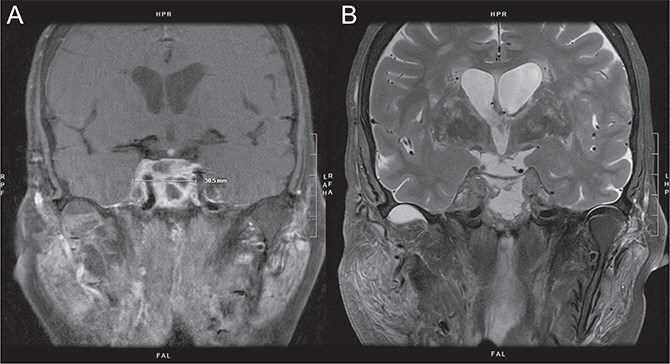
(A and B) MRI brain with contrast, 7 months after initial diagnosis: Heterogenous enhancing sellar mass with involvement of bilateral cavernous sinuses ~23 × 31 × 29 mm.
